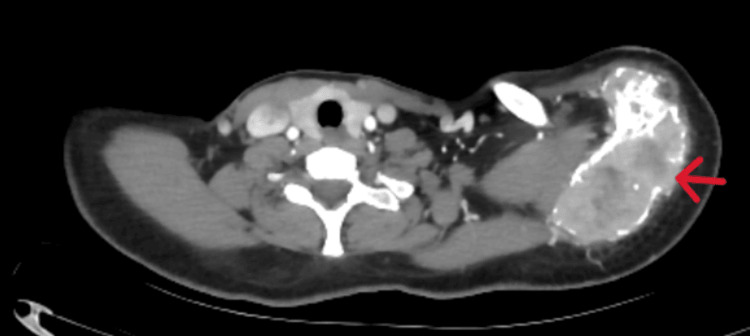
Axial CT scan of the shoulder showing heterogeneous enhancement after contrast injection, with preservation of the vascular bundle.
